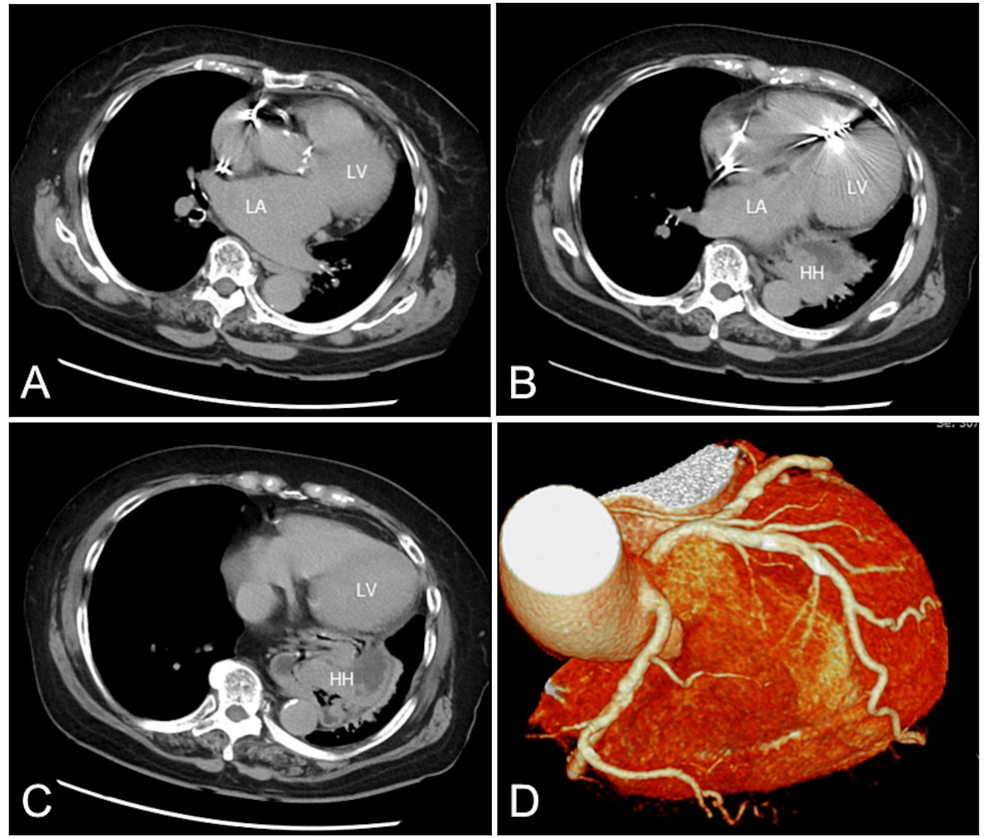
Computed tomographic images Computed tomography revealed calcified aortic valve and a giant hiatal hernia with most of the stomach escaping into the thoracic cavity (A,B,C). No significant coronary atherosclerotic lesions were found (D). The hernia was located behind the LV and LA. There was no significant finding of cardiac compression. LV: Left ventricle; LA: Left atrium
